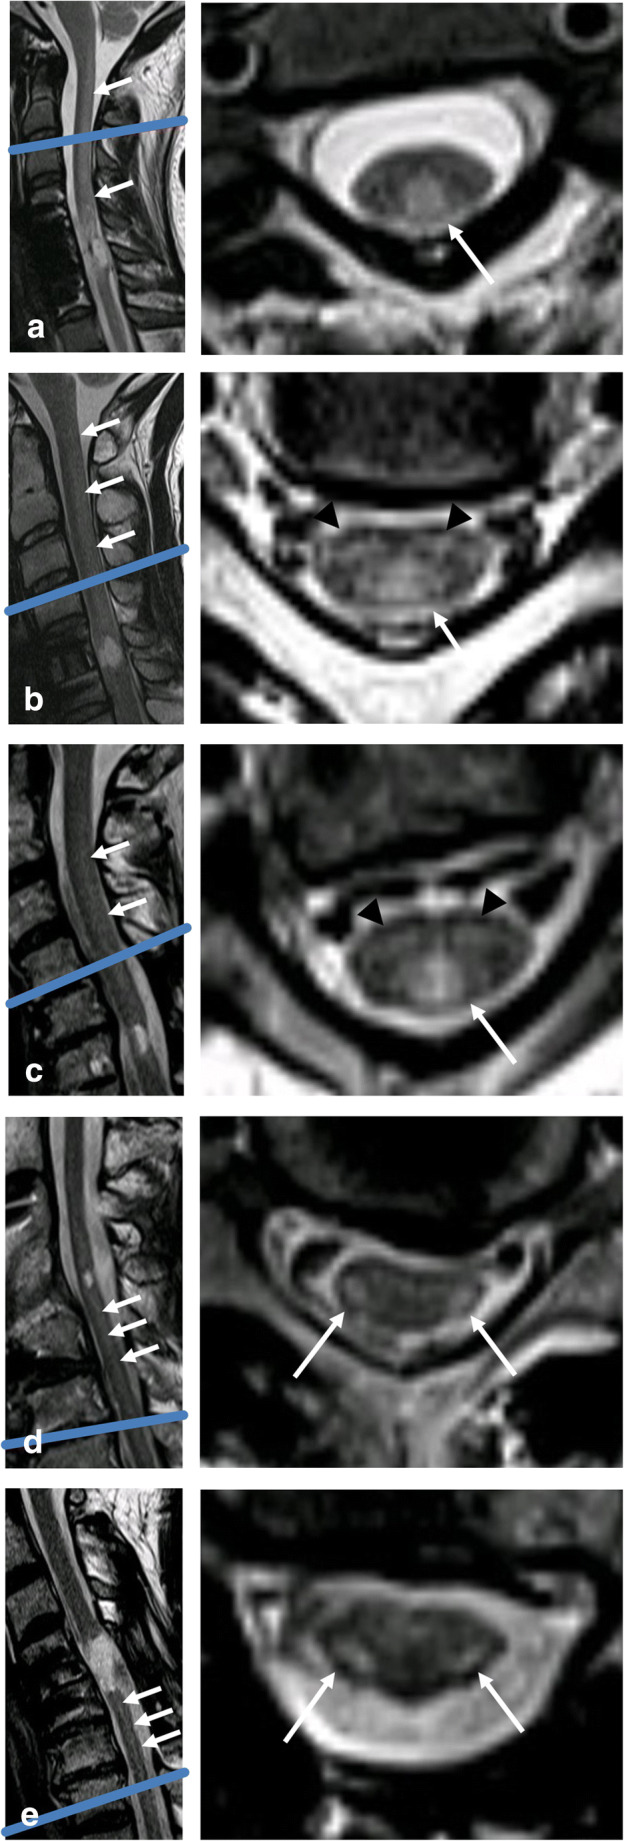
Sagittal and axial T2w MRI of the cervical spine of five different patients demonstrating examples of signal intensity change consistent with Wallerian degeneration (WD) in the dorsal column (DC), the lateral corticospinal tract (CS), and the lateral spinothalamic tract (ST). Axial plane is indicated by the blue line on the corresponding sagittal image. a A 19-year-old patient 348 days after motor vehicle accident (MVA) demonstrating WD in the DC on axial images (white arrow). On median, sagittal imaging, WD (white arrows) can be appreciated from the injury upwards to the medulla oblongata. b A 22-year-old patient 109 days after fall demonstrates WD in the DC on axial and median sagittal imaging (white arrows). In the anterior cord, bilateral WD in the ST is seen (black arrowheads). c A 60-year-old patient 111 days after surfing accident also demonstrates WD in the DC (white arrow) and ST (black arrowheads) on axial imaging. On median sagittal imaging, faint WD in the DC is seen (white arrows). d A 50-year-old patient 323 days after MVA demonstrates WD in the CS (white arrows) on axial imaging. On paramedian sagittal imaging, faint WD in the CS from the injury downwards can be appreciated (white arrows). e A 55-year-old patient 216 days after fall demonstrates WD in the CS on axial and paramedian sagittal imaging (white arrows)
